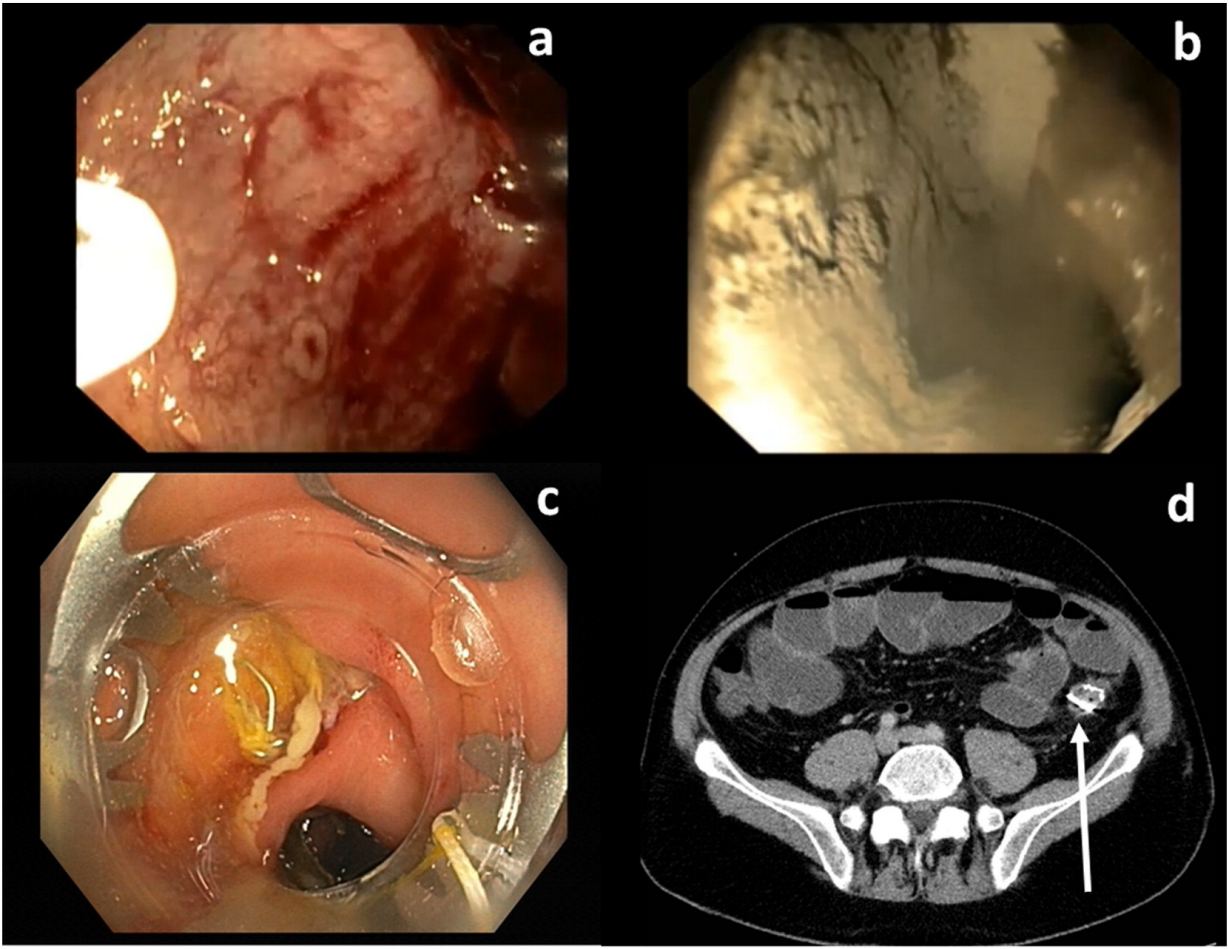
Endoscopic therapy. Through the scope (TTS): a — Duodenal bleeding, Hemospray catheter. b — successful hemostasis after application of hemospray powder. Over the scope clip (OTSC): c — endoscopic view of a postoperative fistula in the colon, OTSC device on the tip of a standard colonoscope. d — Computed tomography scan with OTSC in situ after successful closure of the fistula.
